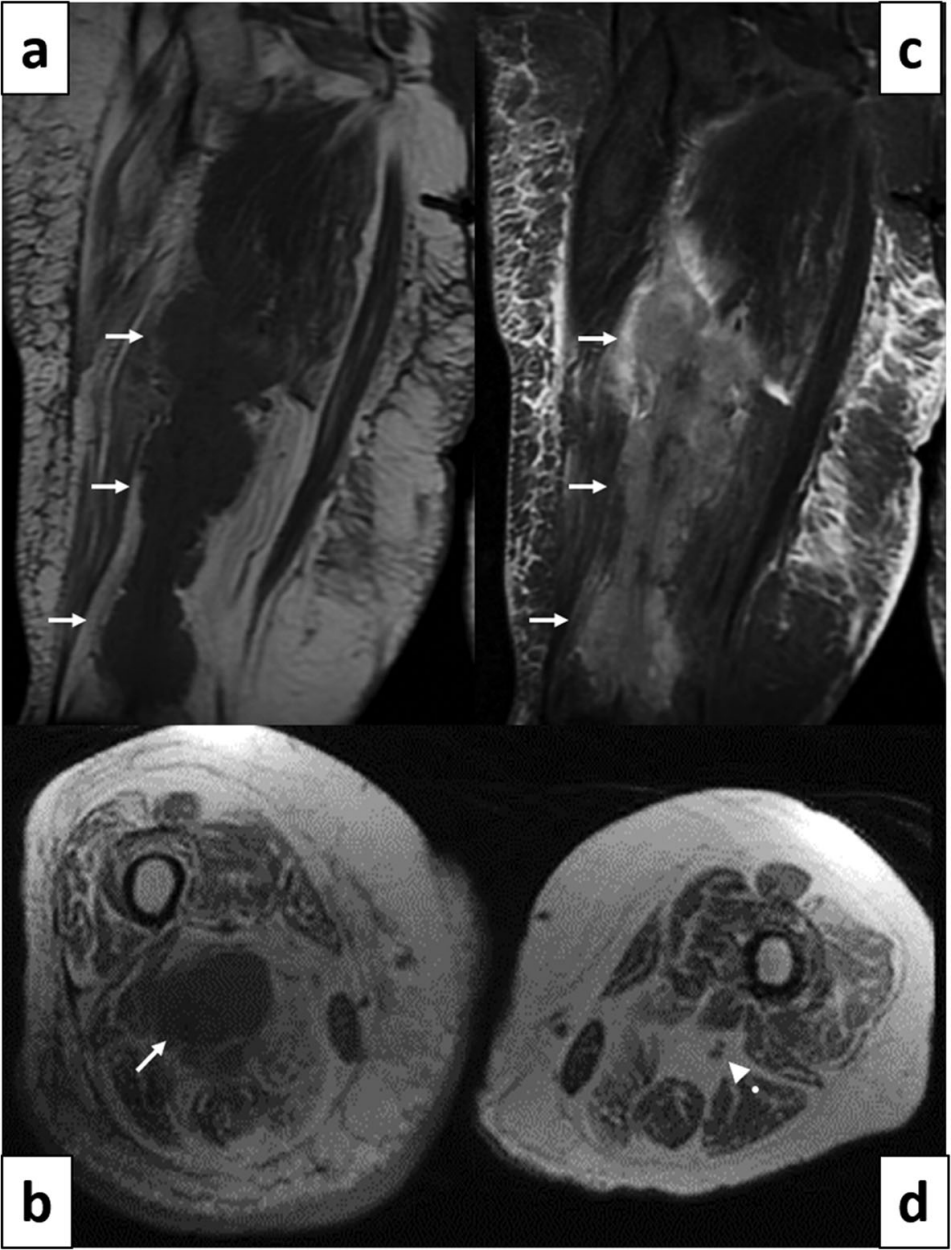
Case 3. 67 years old woman. Diffuse multi-lobular mass along the right sciatic nerve on MRI. a Coronal T1-weighted imaging demonstrating irregular hypointense thickening of the right sciatic nerve (arrows) and diffuse fatty infiltration of the same muscles. b Coronal STIR imaging demonstrating corresponding thickening and increased signal intensity of the sciatic nerve (arrows). c Axial T1-weighted imaging showing thickening of the right sciatic nerve (arrow). The normal contra-lateral sciatic nerve is also observed (arrow head). Please note the marked asymmetry in the diameter of the thighs, right larger than left
